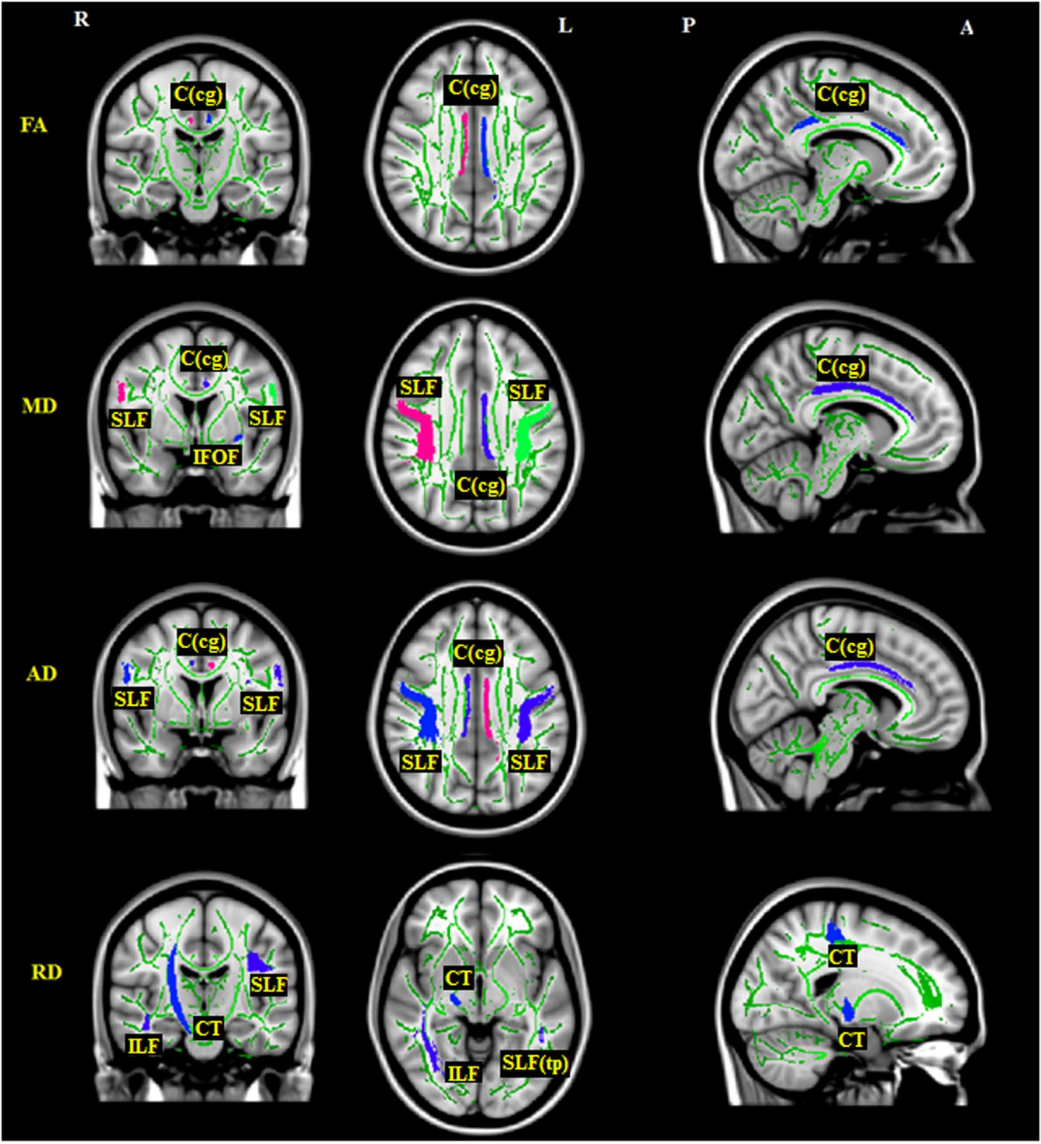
DTI metric differences between participants of Crohn’s disease and healthy control. R: right; L: left; A: anterior; P: posterior. C(cg): cingulum (cingulate gyrus); IFOF: inferior fronto-occipital fasciculus; CT: corticospinal tract; ILF: inferior longitudinal fasciculus; SLF: superior longitudinal fasciculus; SLF(tp): superior longitudinal fasciculus (temporal part).
